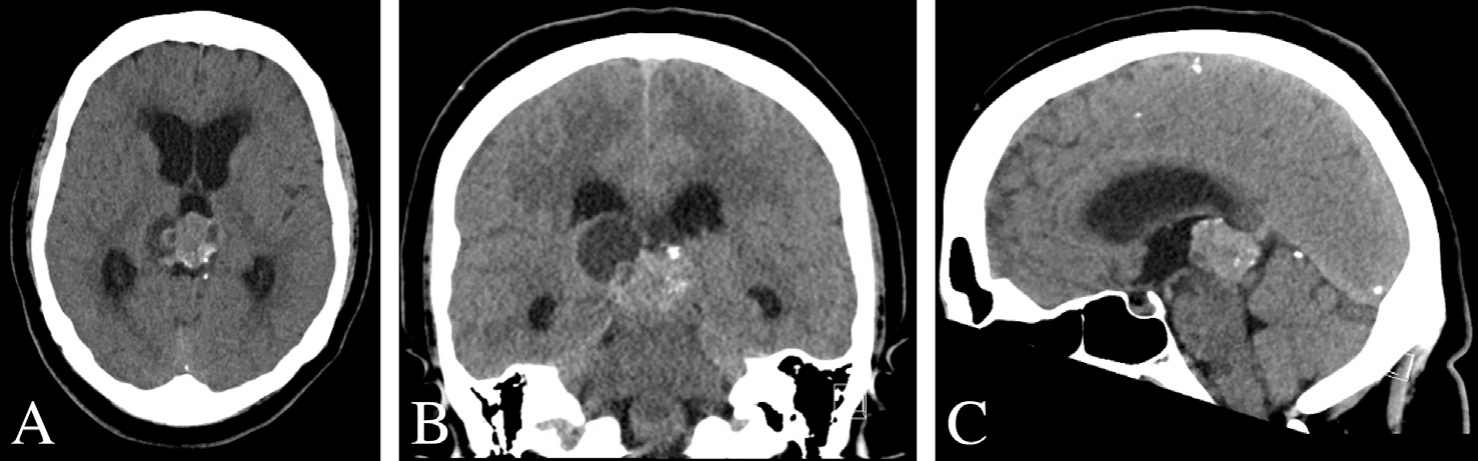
Pre-operative CT scan of the brain. (A–C) Non-contrast CT imaging demonstrating a heterogeneous mass with cystic features and calcification in pineal region causing obstructive hydrocephalus.
